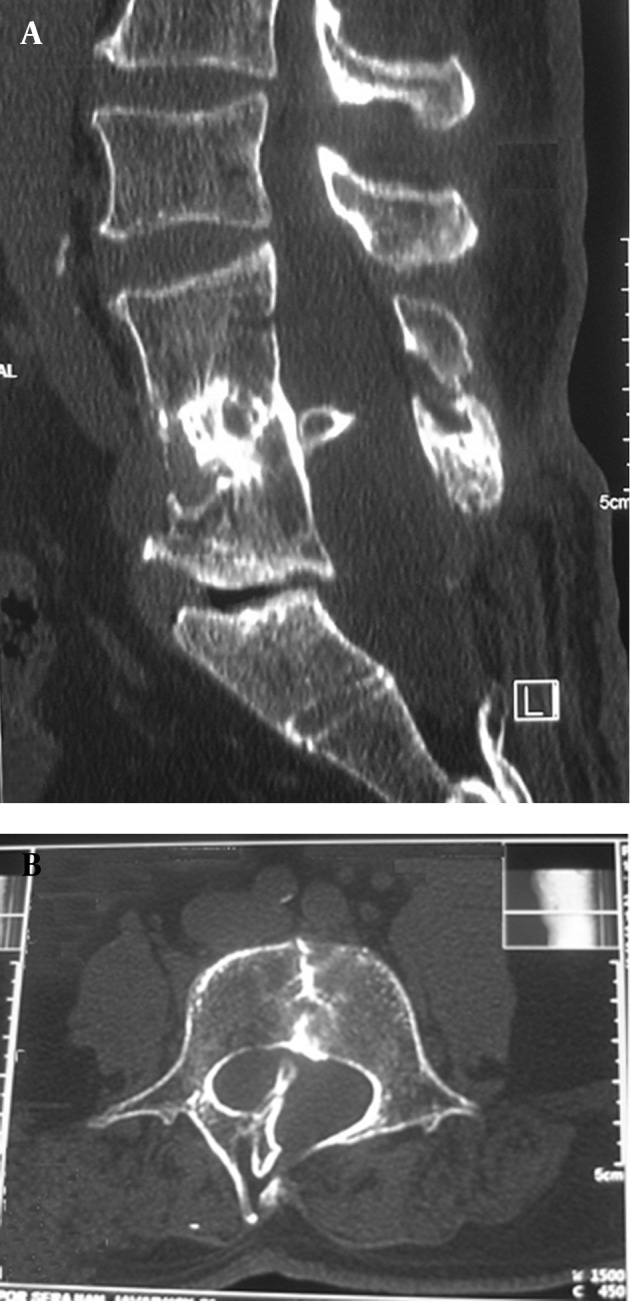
Mid-sagittal (A), and Axial (B) CT Images Showing Bony Spur That Extends to the Posterior Lamina ofL4, Spina Bifida at S1-S2, and Block Vertebra From L3 to L5
